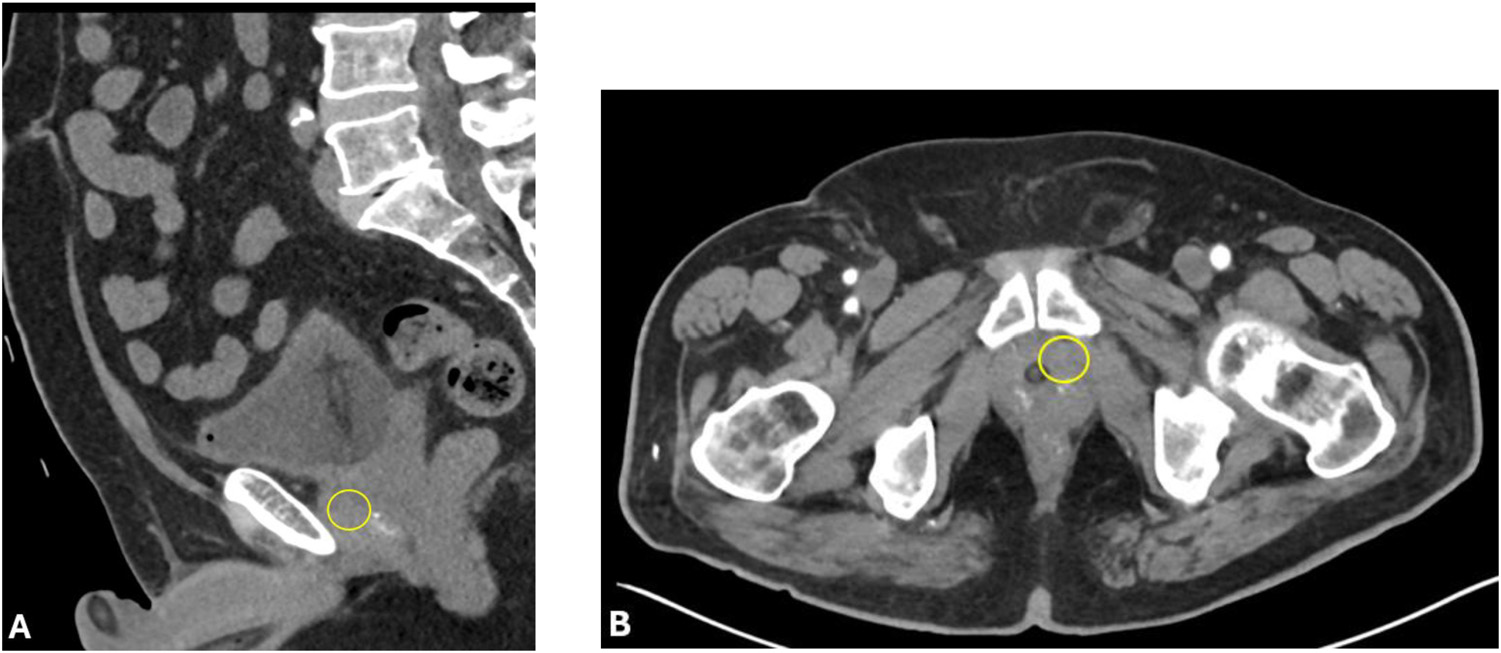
Post-embolization CT angiography demonstrating resolution of pseudoaneurysm. Post-embolization sagittal (A) and axial (B) CT angiography show complete interval resolution of the previously visualized pseudoaneurysm; the yellow circles mark the prior site of the lesion, which is no longer seen following successful embolization.
